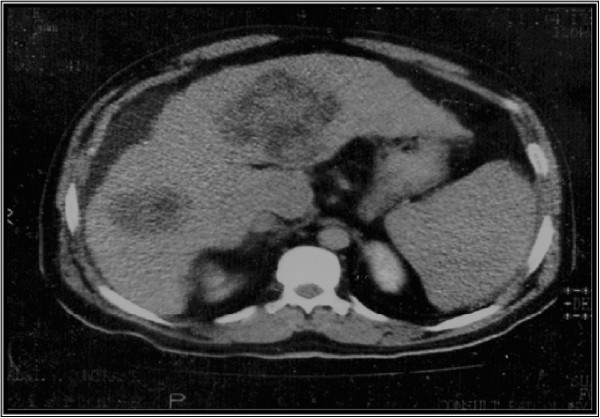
Showing CT scan with HCC of variant size in both lobes of liver.
